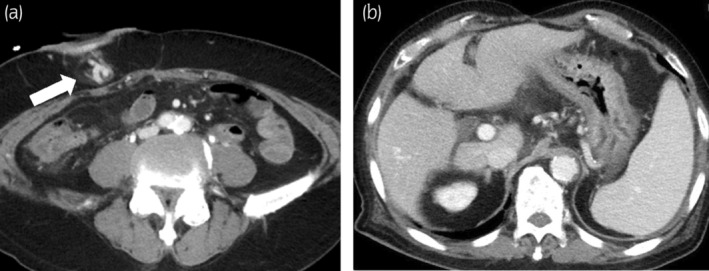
CT images of the mesenteric varices and liver cirrhosis. Images were acquired at the time of hemorrhage (a). The white arrow indicates expansion of the mesenteric vein. CT also revealed liver cirrhosis and splenomegaly (b).
